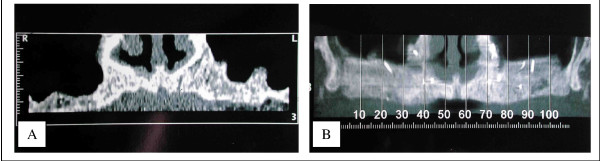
Advantages of the Lefort 1 procedure in case of jaw discrepancy: It provides bone where needed. 8A – CT scan before. 8B – CT scan after.
